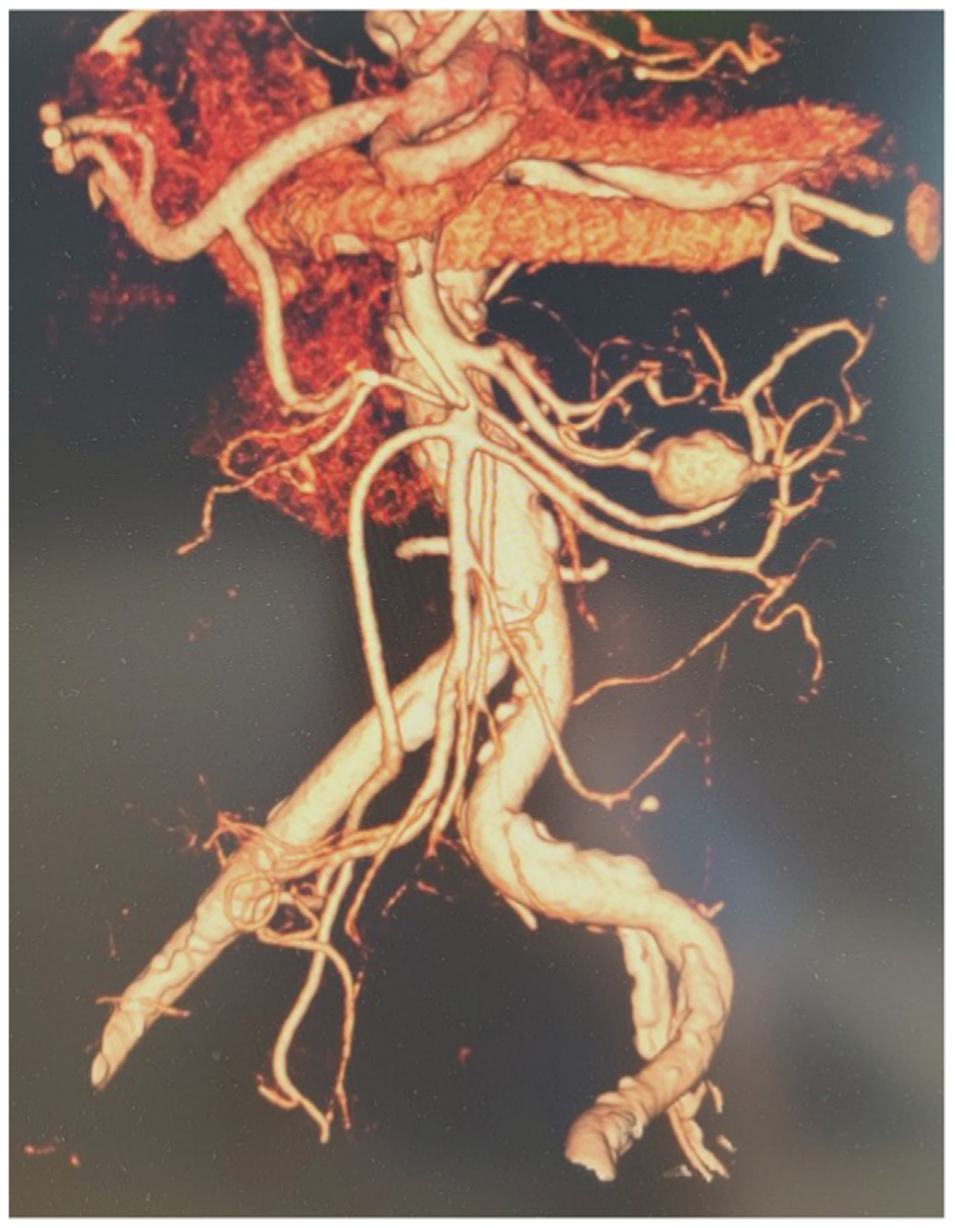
Follow-up computed tomography angiography (CTA) showing a 21-mm jejunal artery pseudoaneurysm with an irregular wall and fat strandings.
